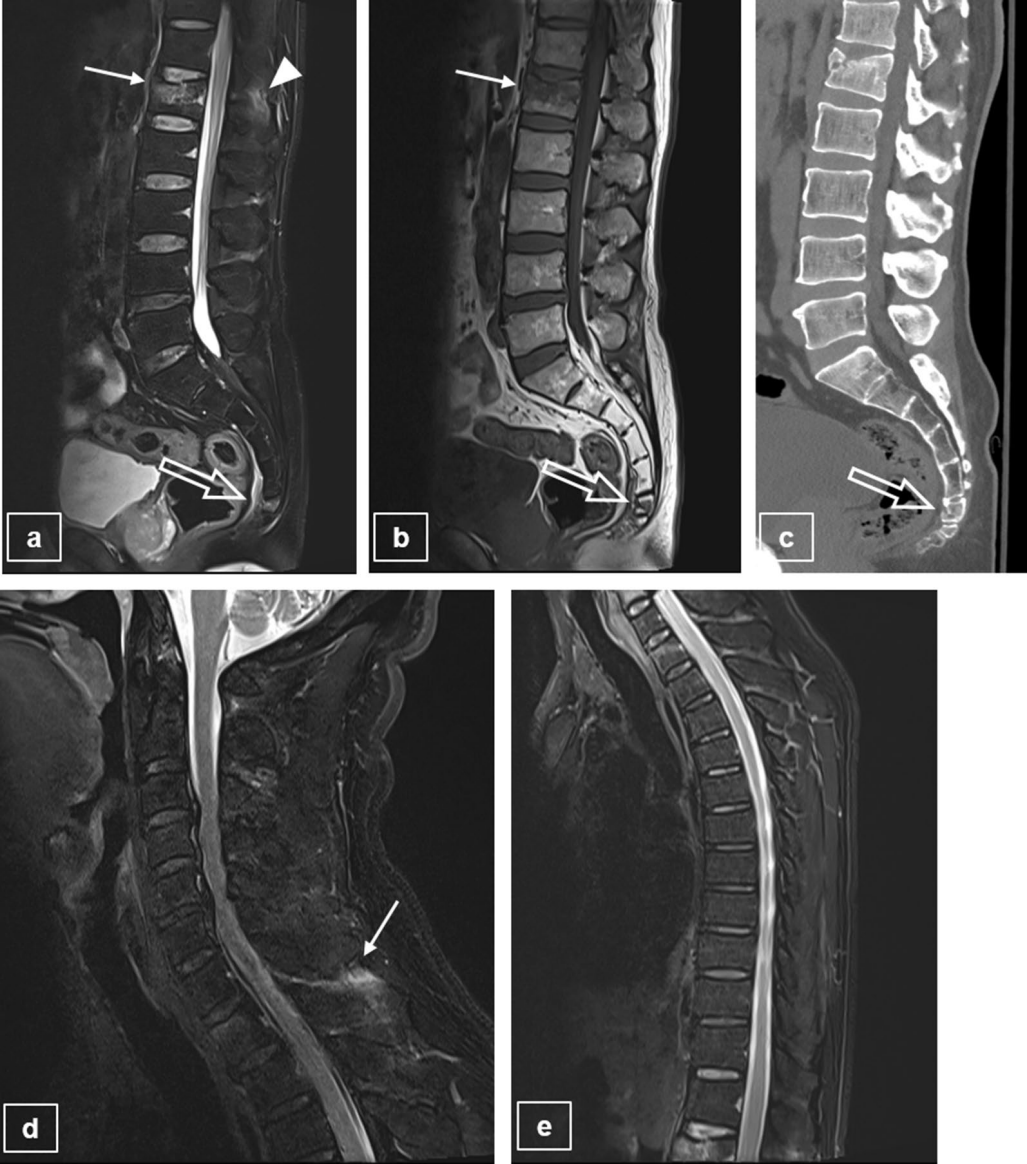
Example of concurrent fractures of the TLJ and SC regions on L-spine MRIs using the modified MRI protocol. Modified L-spine sagittal MRI scans with T2-WI (a) and T1-WI (b) show a recent compression fracture (arrow) at the L1 vertebral body with PLC injury (arrowhead), and a recent fracture at the coccyx upper body (open arrow), which is revealed on a modified L-spine CT scan (c). On scout scans with T2-WI fat saturated sequence of cervical and thoracic spines (d, e), there is no recent fracture, but high signal intensity change is seen in the interspinous ligament of C6/7, indicating ligament injury (arrow). *TLJ* thoracolumbar junction, *SC* sacrococcygeal, *WI* weighed image, *PLC* posterior ligamentous complex.
